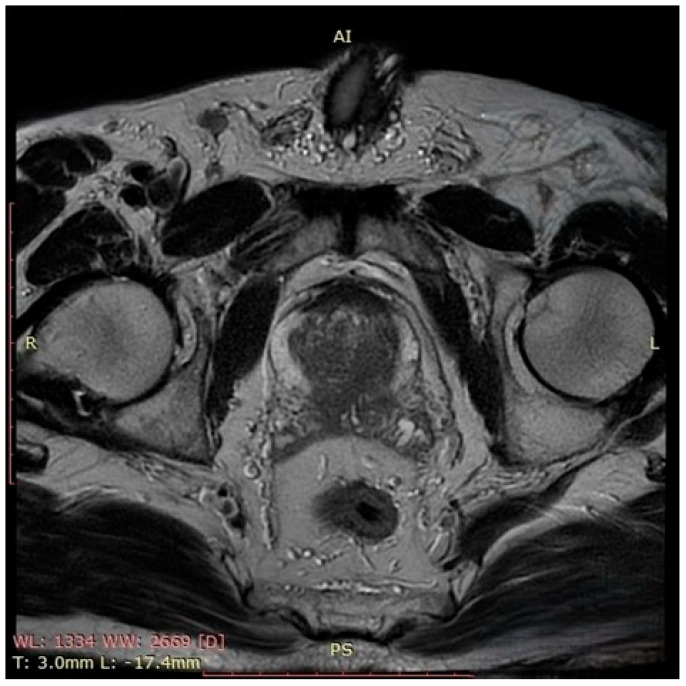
This axial T2-weighted image of the prostate demonstrates a hypointense lesion located in the peripheral zone, bilaterally, which is ill-defined, with disruption of the normal high-signal intensity of the peripheral zone and focal capsular bulging. There is no clear evidence of extracapsular extension or seminal vesicle invasion. These findings are suggestive of a PIRADS 5 lesion and highly suspicious for clinically significant prostate cancer.
